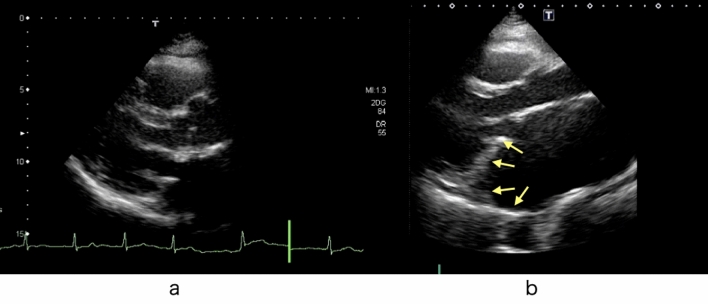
Postoperative echocardiography in a patient with atrial functional MR. a Immediately after the operation. b Twelve years after the operation. The left atrium was enlarged and the posterior mitral annulus was elevated by the enlarged atrial wall
